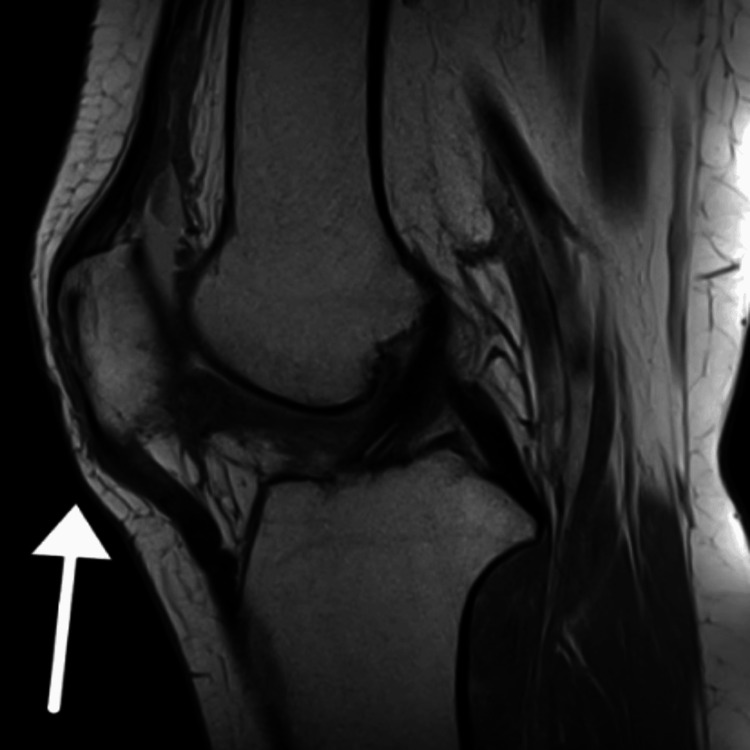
Post-Operative MRI Sagittal view of the knee The area highlighted by the arrow represents the location of the graft, showing improved articular contour. The appearance of normal bone marrow signal intensity around the graft suggests favorable healing, which aligns with the minimal joint effusion observed.
